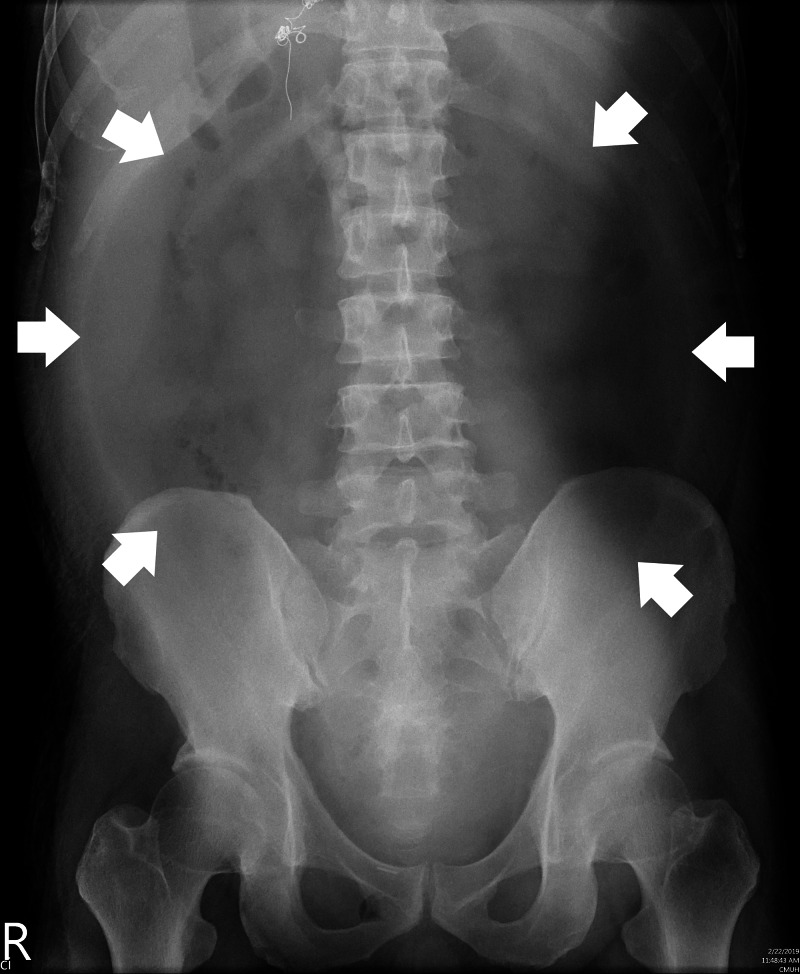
Football sign Football sign in a patient with acute abdominal pain. A vertically oriented oval radiolucency in the central part of the abdomen (white arrows) indicating pneumoperitoneum can be seen on this supine abdominal x-ray.
